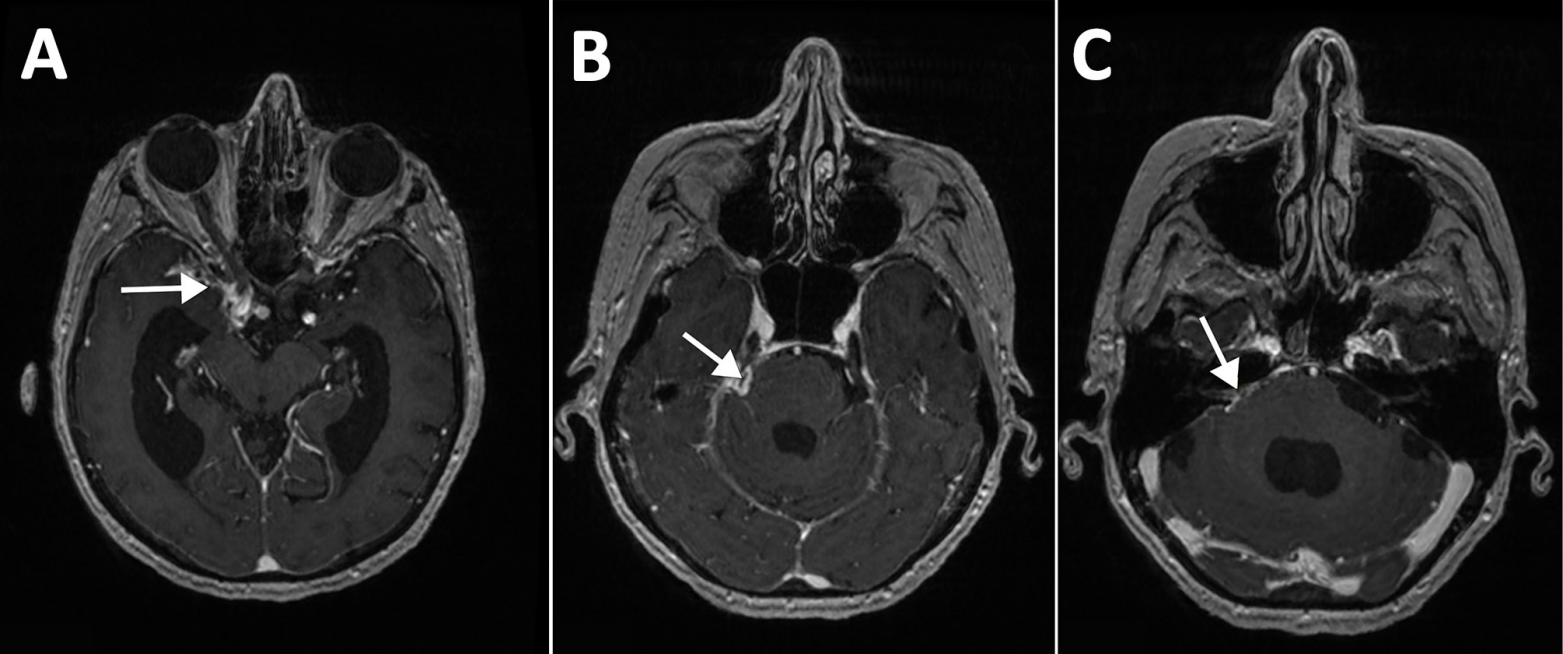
Magnetic resonance imaging of the brain in study of subarachnoid neurocysticercosis caused by larval-stage *Taenia crassiceps* tapeworm, Slovenia. Imaging shows pathological signal enhancement at the site of inflammation after contrast administration (white arrows) and a dilated ventricular system (hydrocephalus) as a result of impaired cerebrospinal fluid drainage in the right basal cisterns (A), right parapontine basal cistern (B), and right pontocerebellar angle (C).
